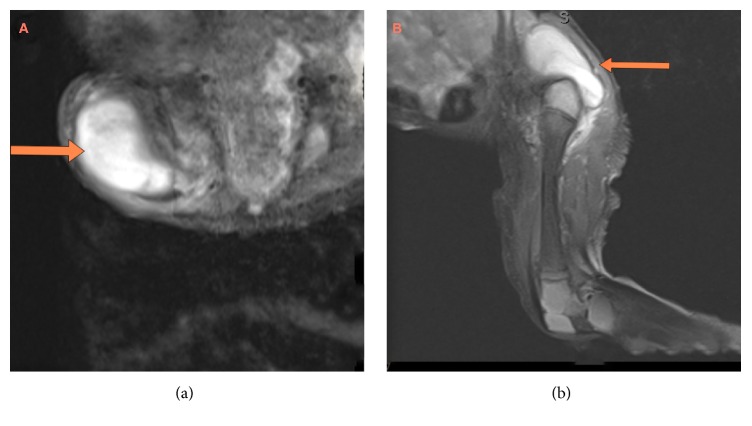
(a) MRI image axial cut demonstrating large subcutaneous fluid collection (arrow) in the thigh and (b) MRI image sagittal cut of the same patient's right hip showing same fluid collection with corresponding joint effusion (arrow) concerning for septic arthritis.
